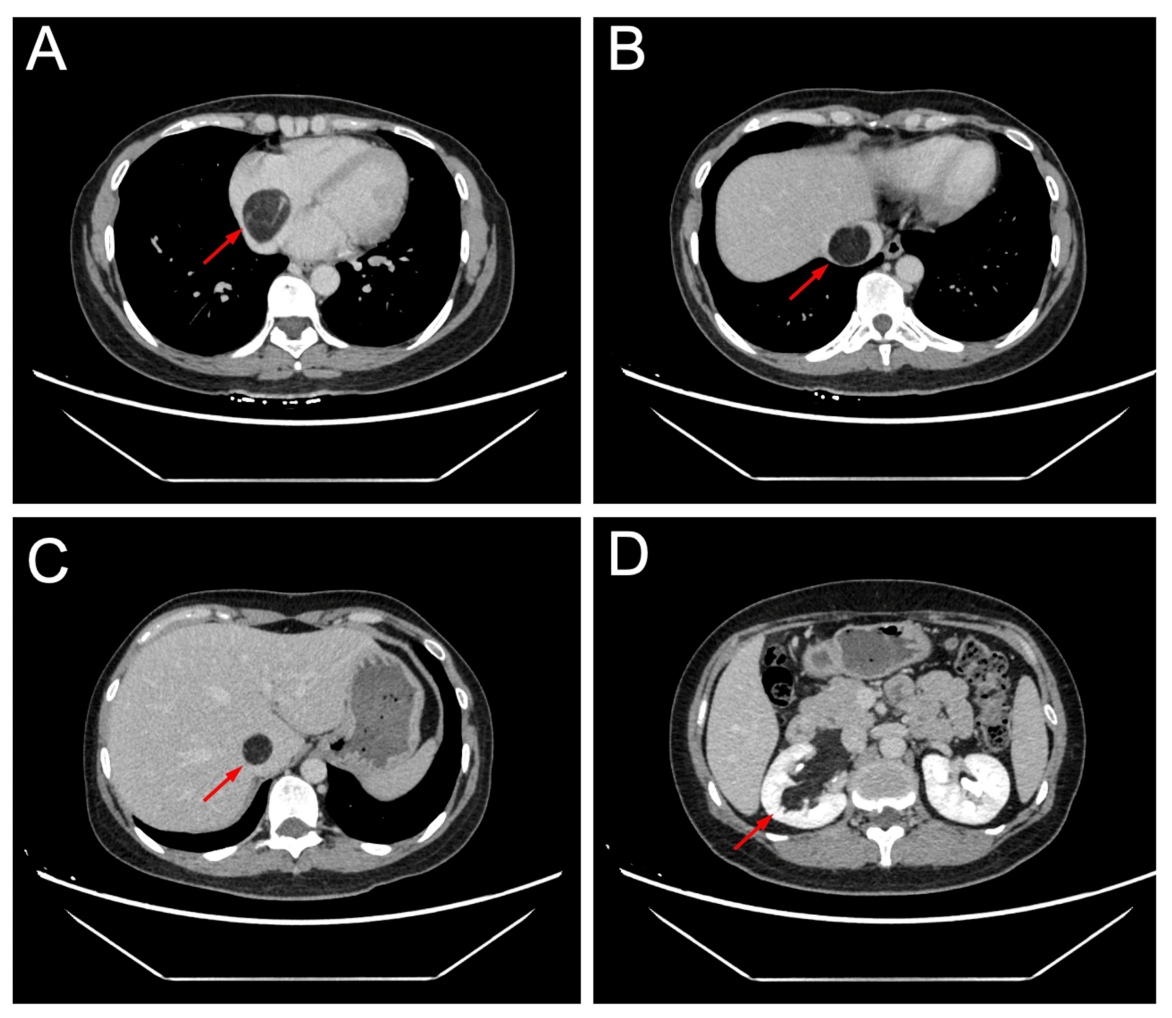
Axial views of the chest and abdominal computed tomography (CT) scan of the patient on admission. The axial views of CT scan showing hypodense masses in the right atrium (A), inferior vena cava (IVC) (B, C), and right kidney (D). (Arrows indicated the masses)
